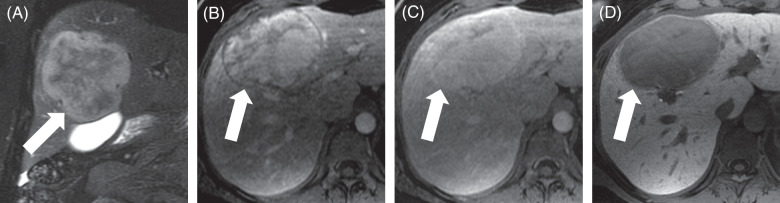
Hepatic adenomas—Characteristic features on MRI with contrast. A 35-year-old woman with metabolic syndrome and an inflammatory hepatocellular adenoma (arrow) undergoes MRI with hepatobiliary contrast. (A) Coronal T2-weighted images show a lesion with moderate T2 hyperintensity that is most pronounced at the periphery, also known as an atoll sign. (B) After injection of contrast, axial T1-weighted images in the arterial phase show that the lesion has heterogeneous hyperenhancement. (C) In the portal venous phase, the enhancement persists slightly above the liver. (D) In the hepatobiliary phase, the mass is hypointense compared to the uninvolved liver.
